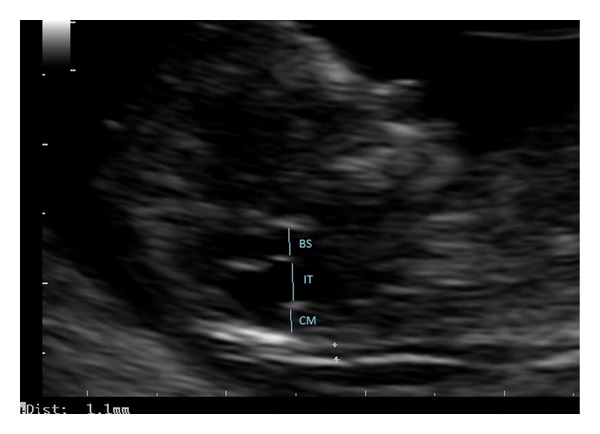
Fetal ultrasound image (sagittal view) at 11 + 4 weeks of gestation, showing an enlarged intracranial translucency (IT) in the posterior fossa, the brain stem (BS), and the cisterna magna (CM).
